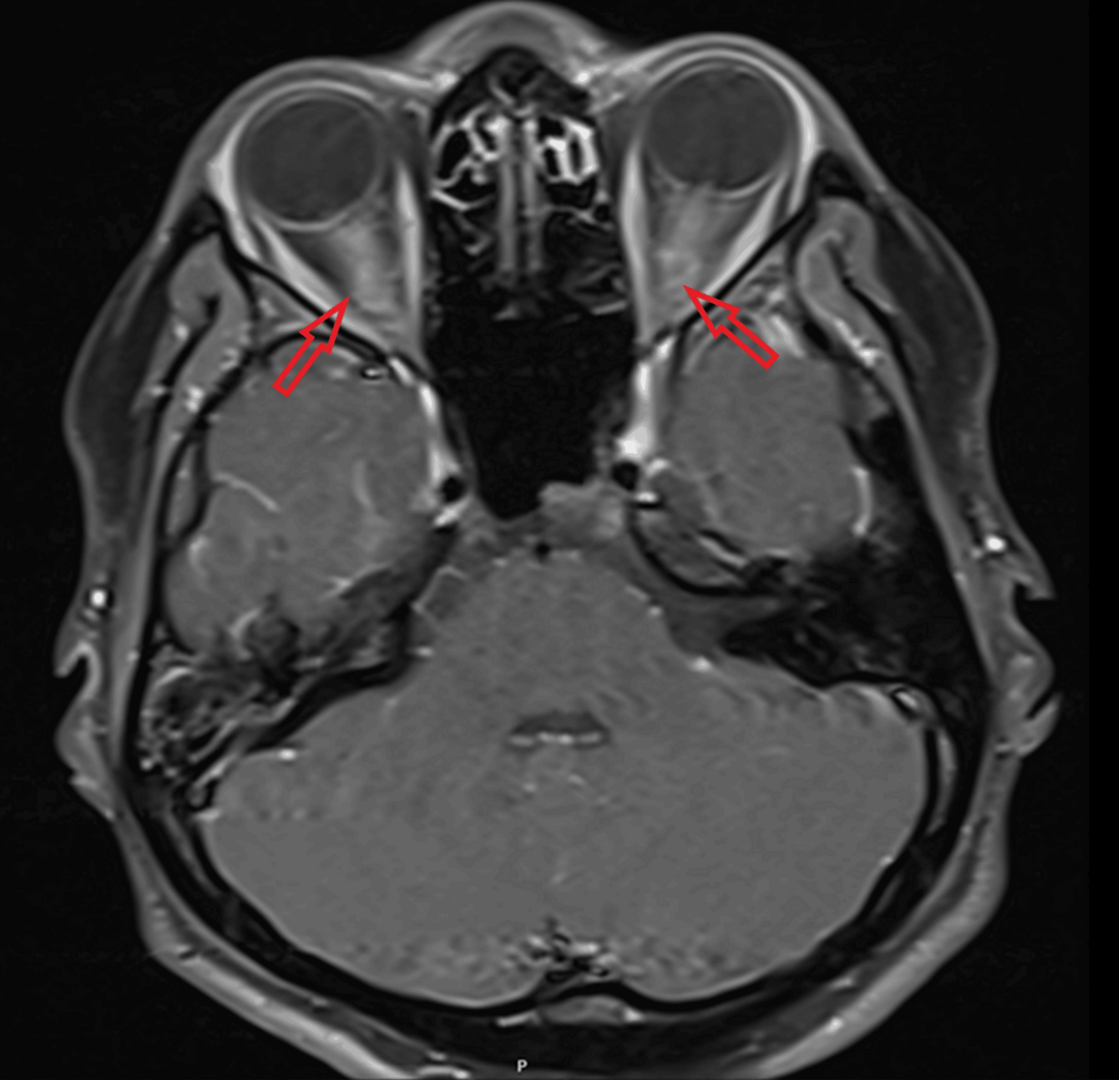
Axial T1 post-contrast MRI showing enhancement (bright signal) around both optic nerve sheaths, indicating inflammation.
